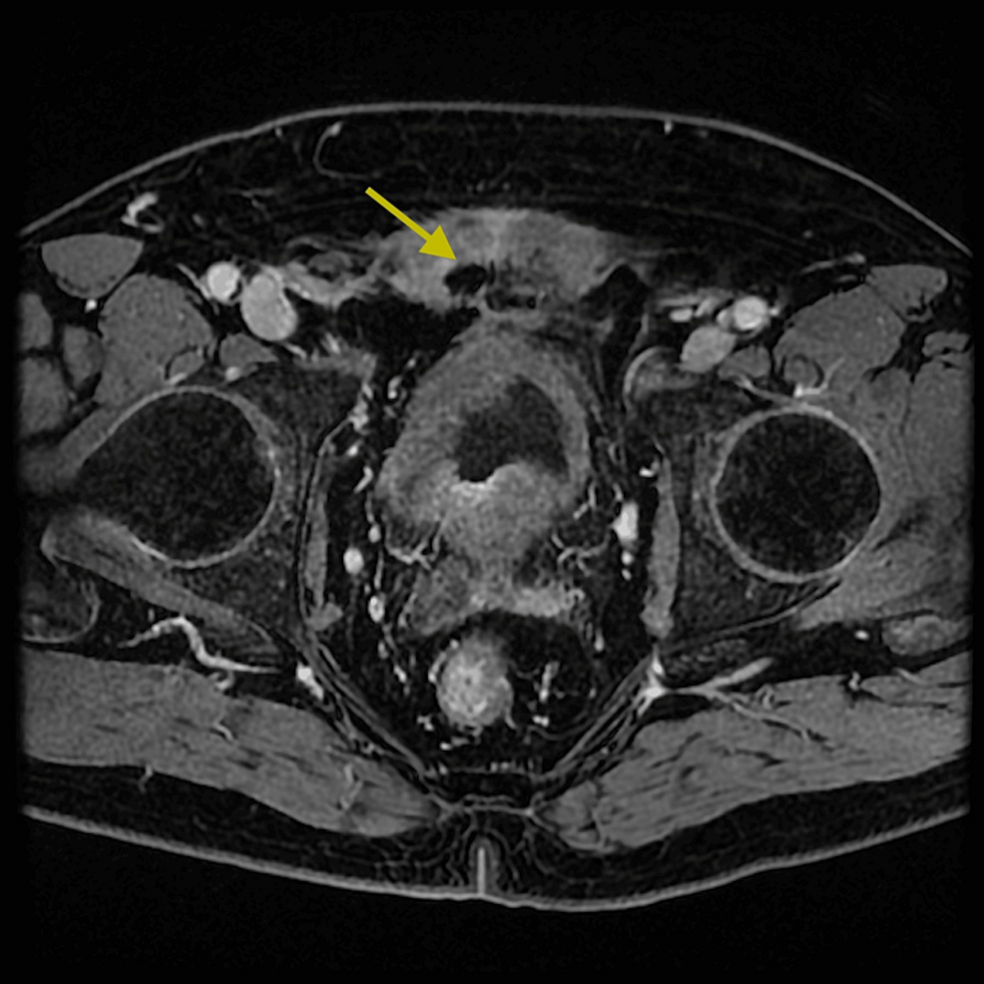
Axial pelvic MRI scan Multiple small abscesses within the Retzius space (arrow).
